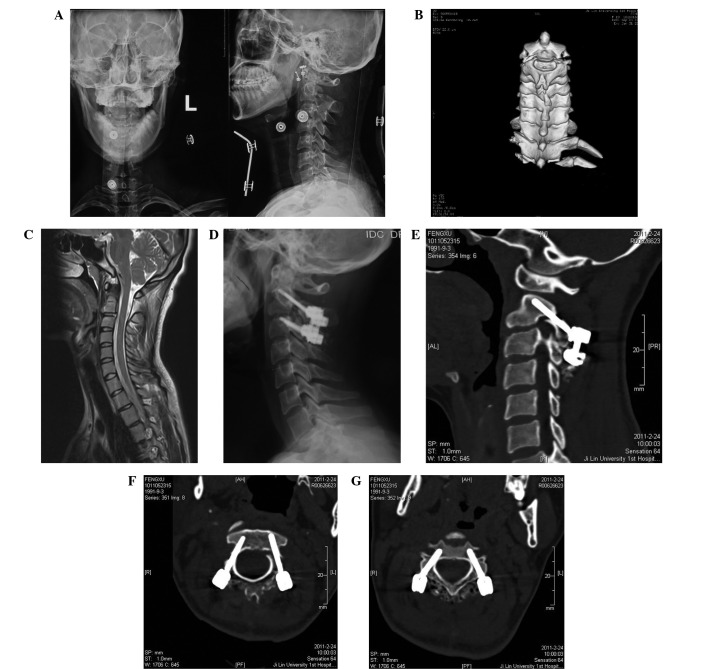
Hangman’s fracture type I. (A) X-ray film showing a type I fracture; (B) three-dimensional CT scan showing the fracture form; (C) MRI scan showing the C2-C3 disc damage at the back and anterior lower margin of the C2 fracture; (D) postoperative lateral X-ray film; (E) postoperative vertical CT; (F) transverse C2; and (G) transverse C3. CT, computed tomography; MRI, magnetic resonance image.
